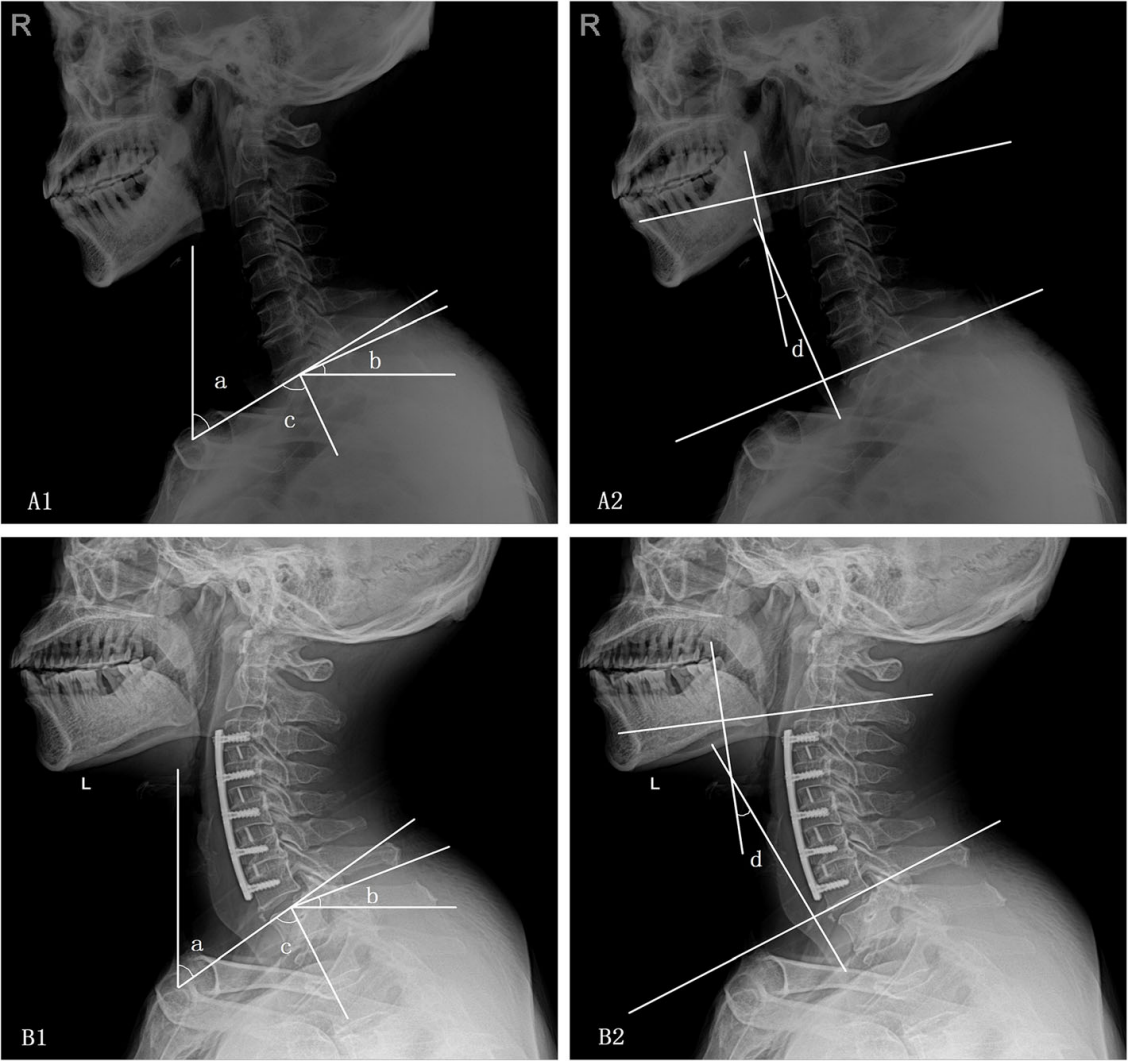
Lateral radiographs of the cervical spine in patients at baseline and at 3 months postoperatively
